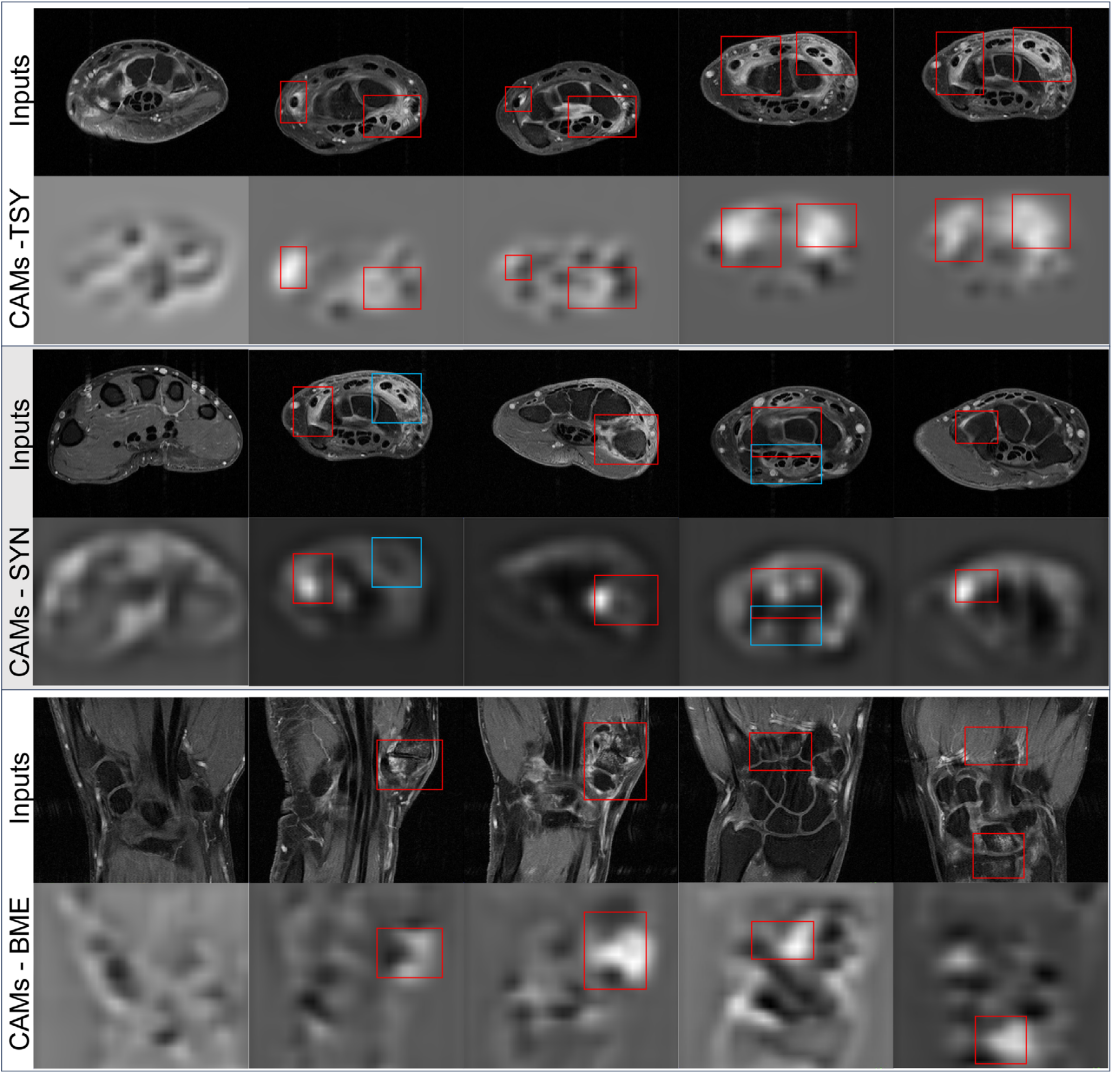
Examples of slices from the CAMs of 15 patients generated from the DL models for estimating total inflammation directly (Route1). Red boxes in the examples highlight the inflammation regions in the MRI scans that are the target regions for inflammation assessment; blue boxes highlight examples of inflamed regions that belong to another category (e.g., tenosynovitis regions when the CAMs were generated from the DL models for synovitis estimation). CAMs are displayed as separate images instead of the commonly used overlays on the original images, in order to allow for an accurate interpretation by clearly distinguishing class activations from pixel intensities.
